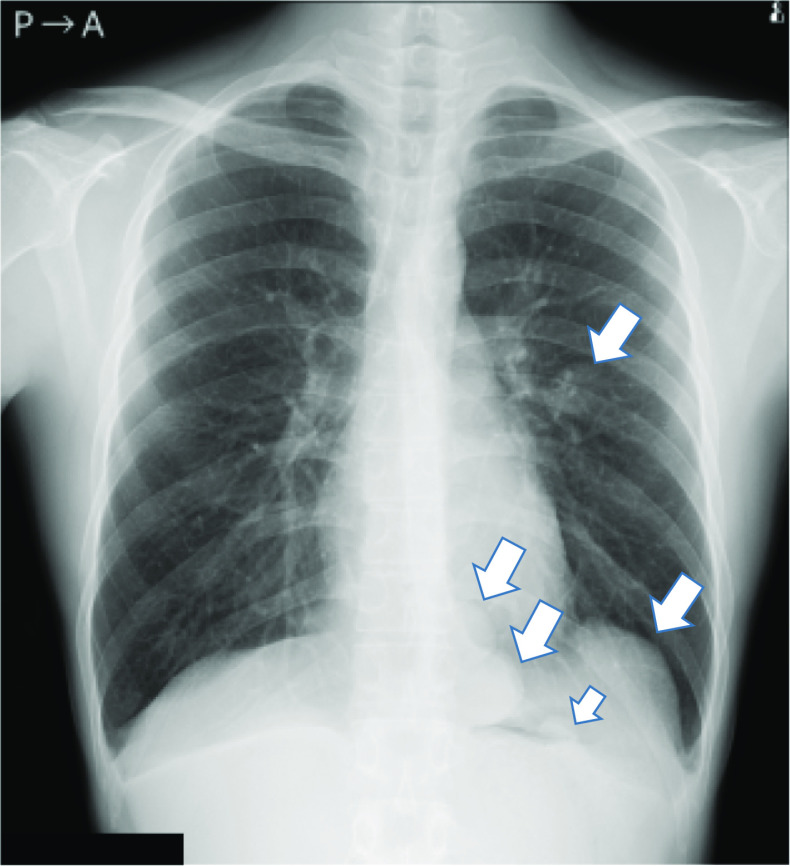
A chest radiograph showed several lesions in the left lung field (arrows)
